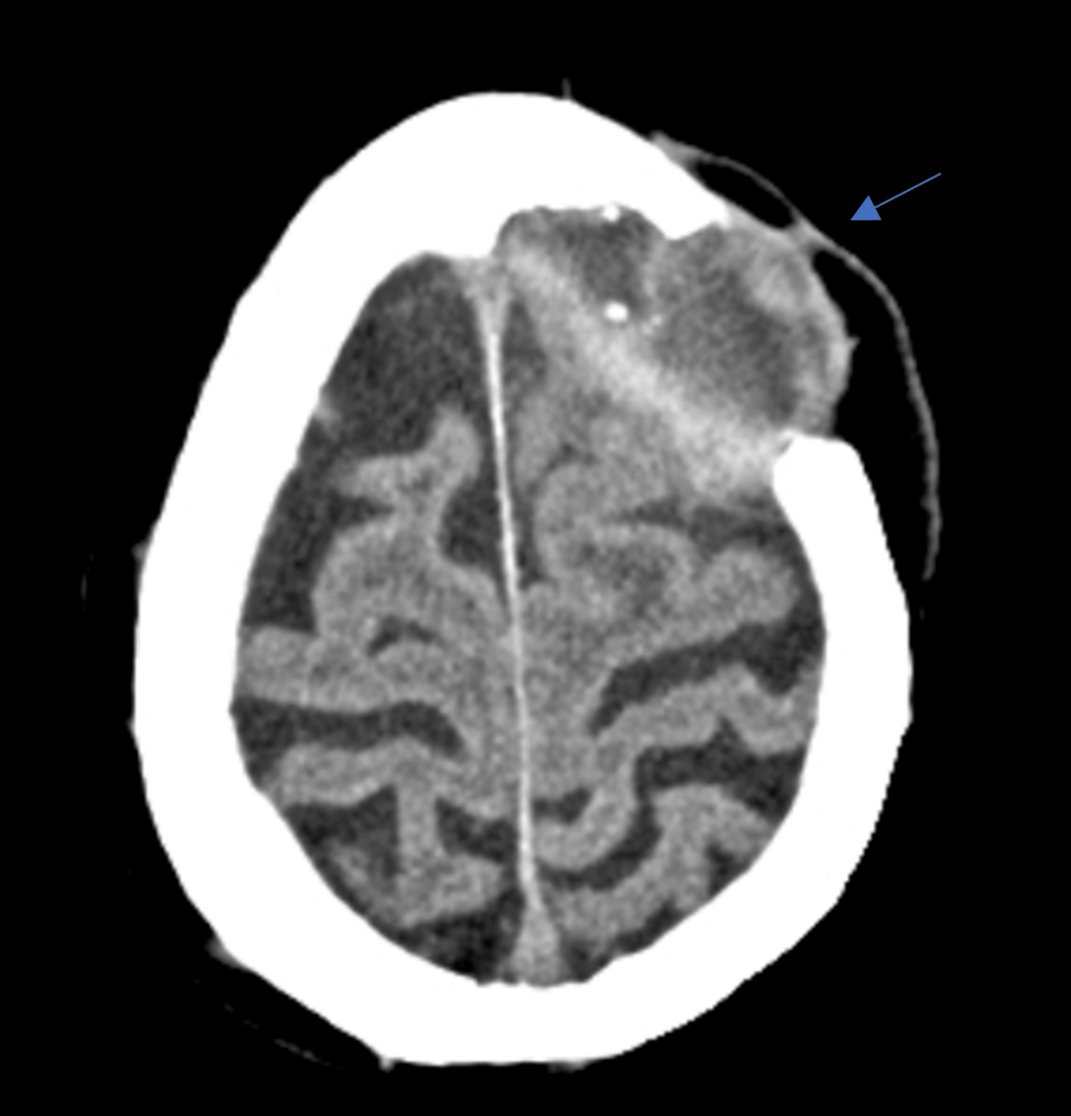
Staging CT scan. CT scan from April 2020 revealing a right frontoparietal lesion with parietal bone invasion and intracranial extension. No distant metastasis was seen.
